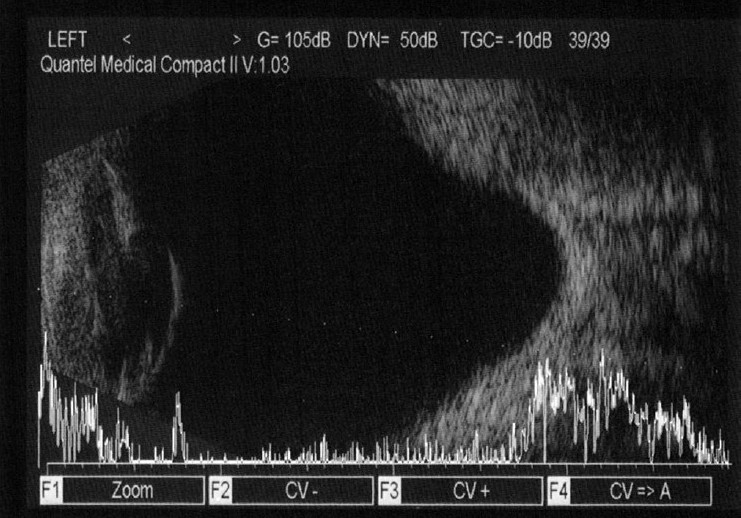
A/B-scan ultrasonography of an eye with posterior staphyloma in the study group
